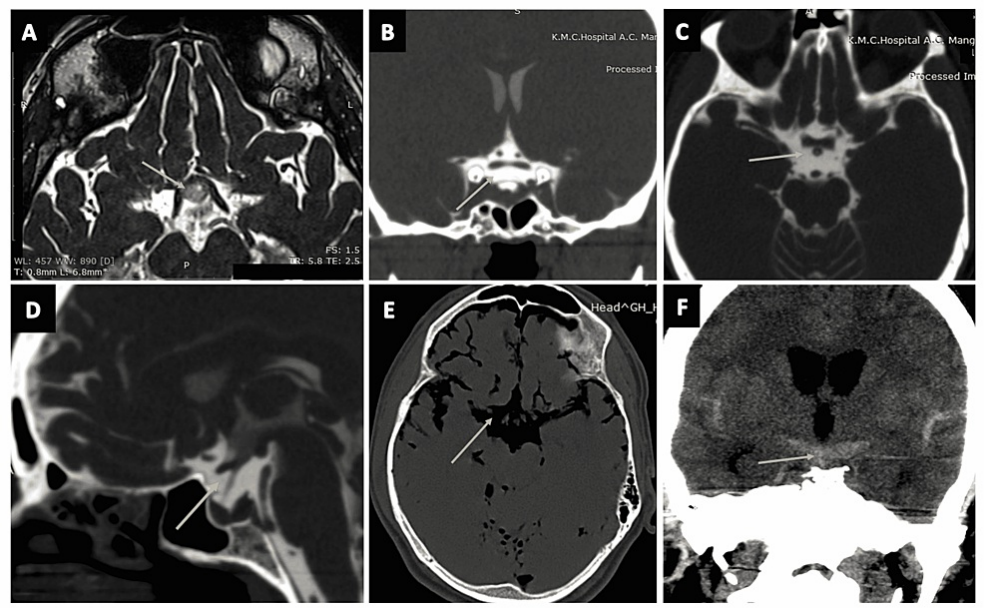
The cisterna chiasmatica (chiasmatic cistern). (A) The chiasmatic cistern (arrow) as seen on an axial section of the routine three-dimensional (3D) constructive interference in steady state (CISS) MRI sequence. (B), (C), and (D) show the chiasmatic cistern (arrows) as seen on the coronal, axial, and sagittal sections of CT cisternography, respectively, in a case of suspected CSF rhinorrhoea. (E) The chiasmatic cistern (arrow) visualized in the axial section of CT brain (negative contrast) in a case of pneumocephalus. (F) The chiasmatic cistern (arrow) as visualized on the coronal section of CT brain (positive contrast) in a case of subarachnoid hemorrhage (SAH).
